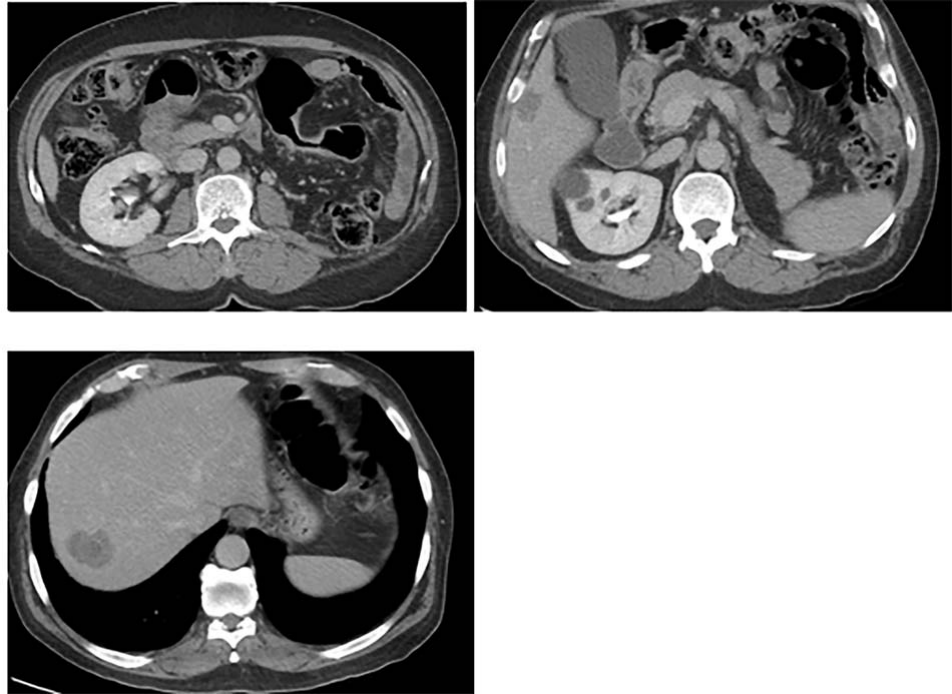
CT: status of post administration of NAC.
